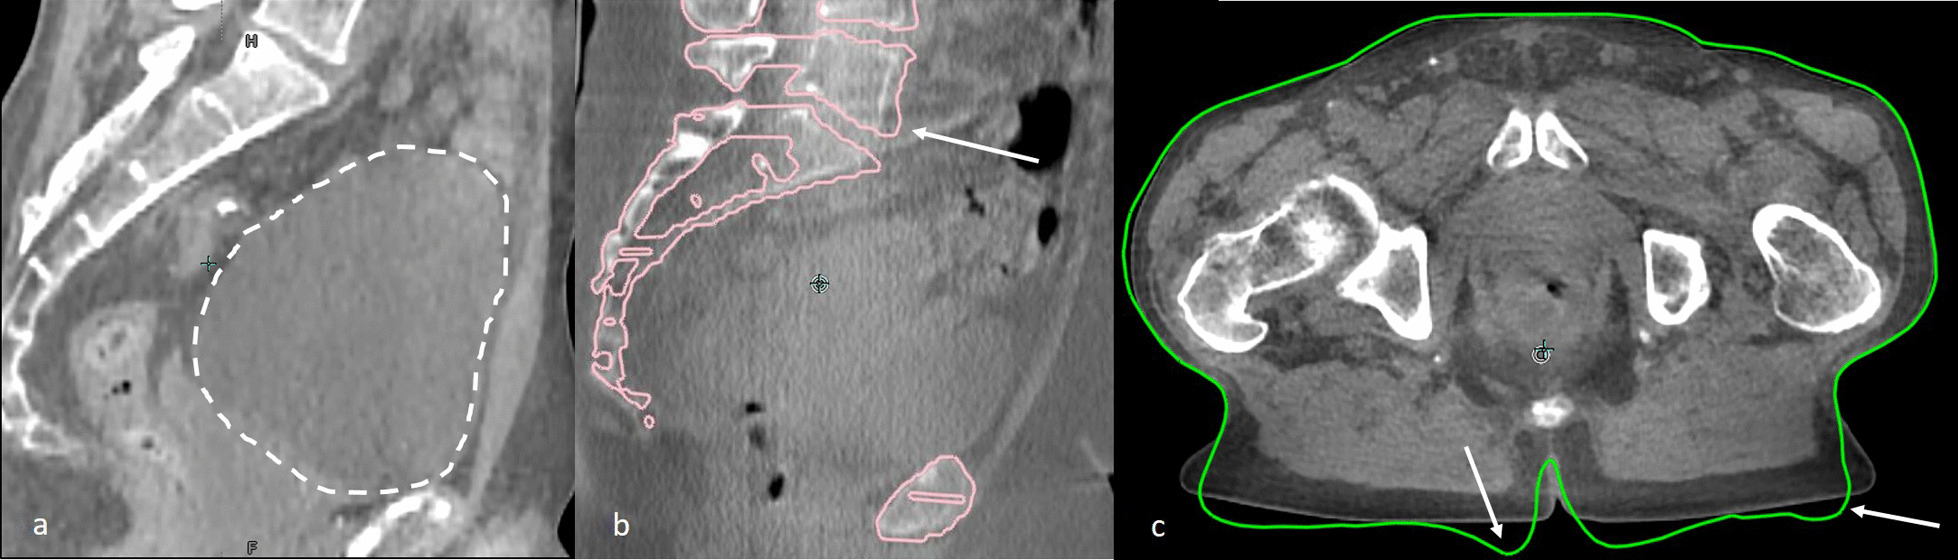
CBCT with a too full bladder at start of treatment (a). A small error with respect to deformable bony anatomy registration of CT to CBCT. In pink the representation of the bony anatomy of the sCT with overlay on the CBCT (b). A small error with respect to deformed body contour of CT to CBCT. In green the representation of the body contour of the sCT with overlay on the CBCT (c)
